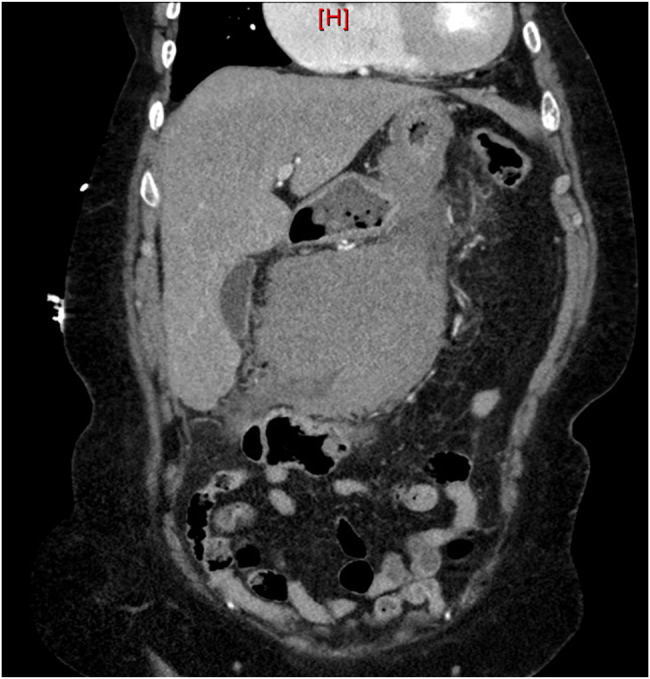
Computed tomography of the abdomen/pelvis with intravenous contrast: large exophytic mass arising from the greater curvature of the stomach. Gastrointestinal stromal tumor favored.
